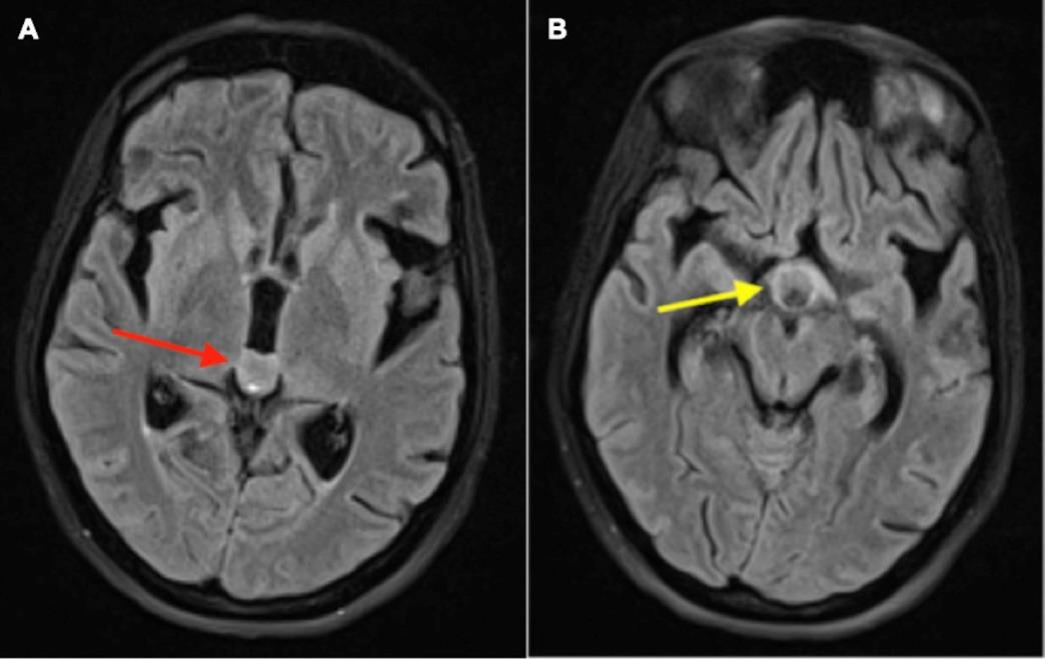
Brain MRI images showing mildly bulky pineal gland (A), bulky pituitary infundibulum (B)
